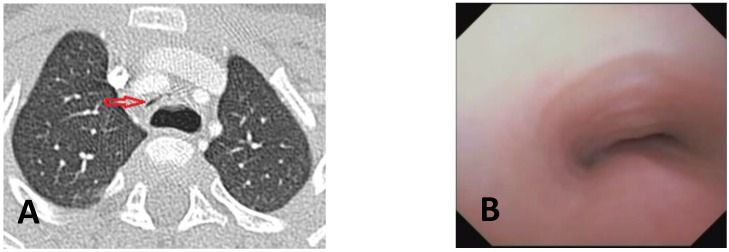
(A) Chest CT with CM: Tracheal lumen completely collapsed (arrow) at the level of anterior compression by the IA and posteriorly by the dilated esophagus. (B) SVBS: Endoscopic view of the trachea above the TEF: Tracheal lumen almost completely collapsed even during quiet breathing. Abbreviations. CT: Computed Tomography; CM: Contrast Medium; IA: Innominate Artery; SVBS: Static Videobronchoscopy.
